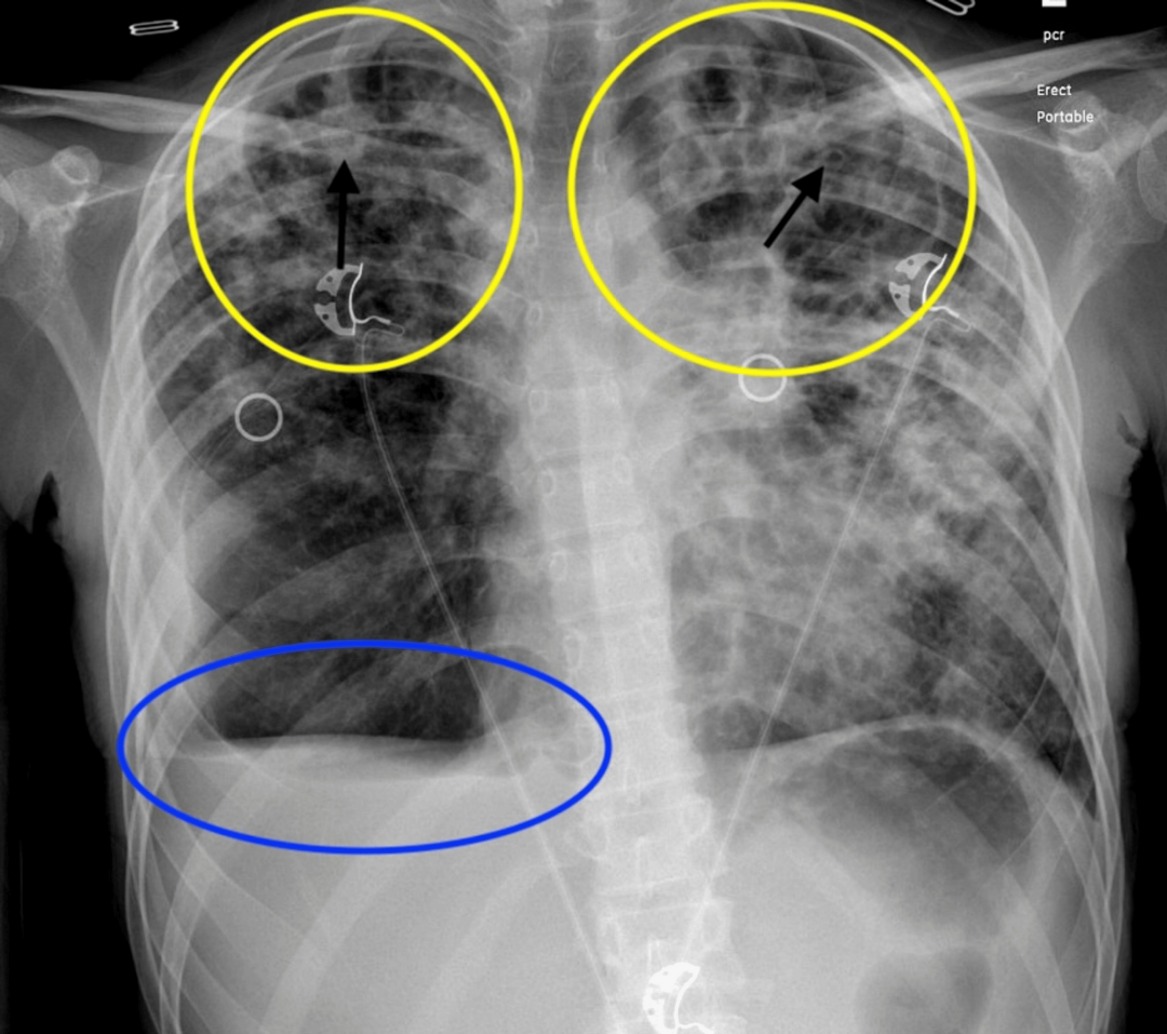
Chest X-ray demonstrating bilateral upper lobe ground glass opacities (yellow circles) with interlobular and peribronchial thickening (black arrows) alongside pleural effusion with blunting of the costophrenic angle (blue oval).
